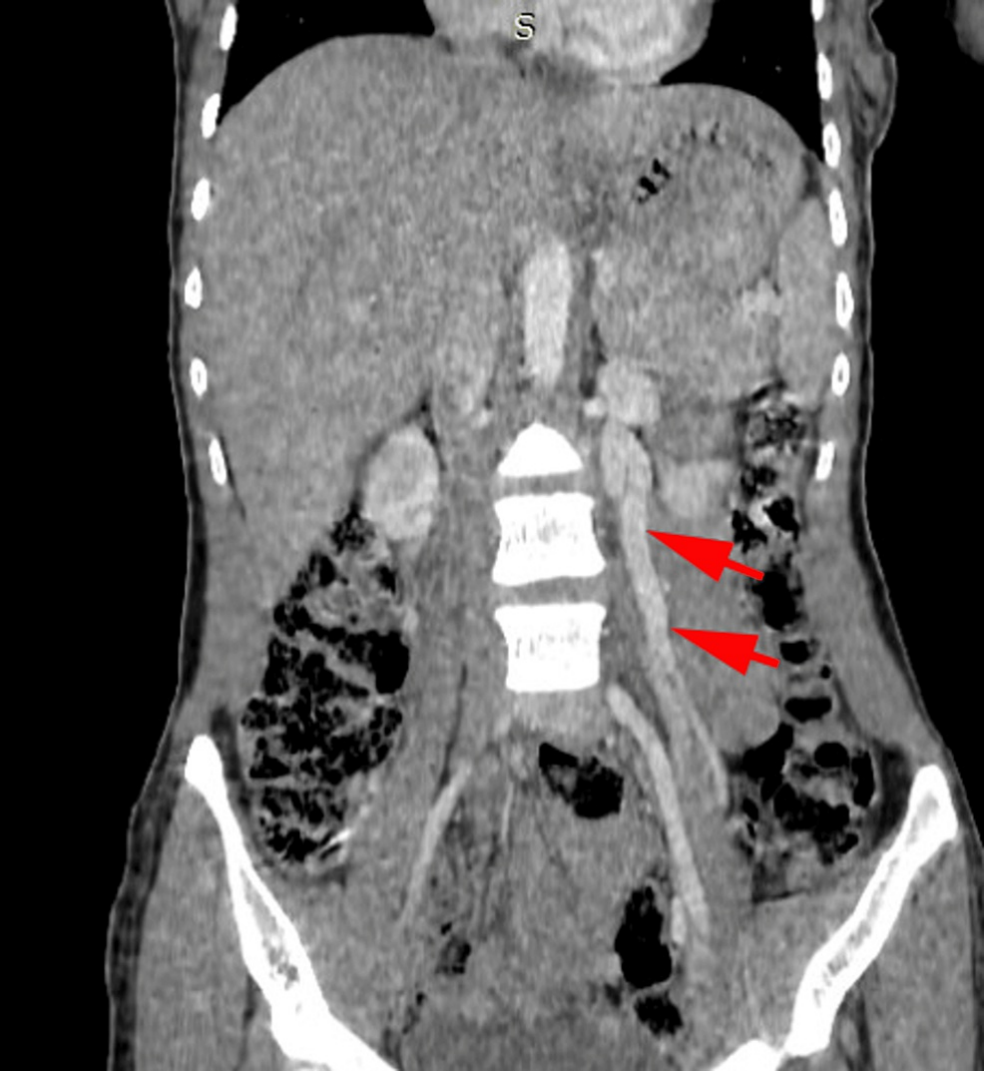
CT scan of the abdomen and pelvis (coronal view) The image is showing dilation of the left ovarian vein (red arrows).
